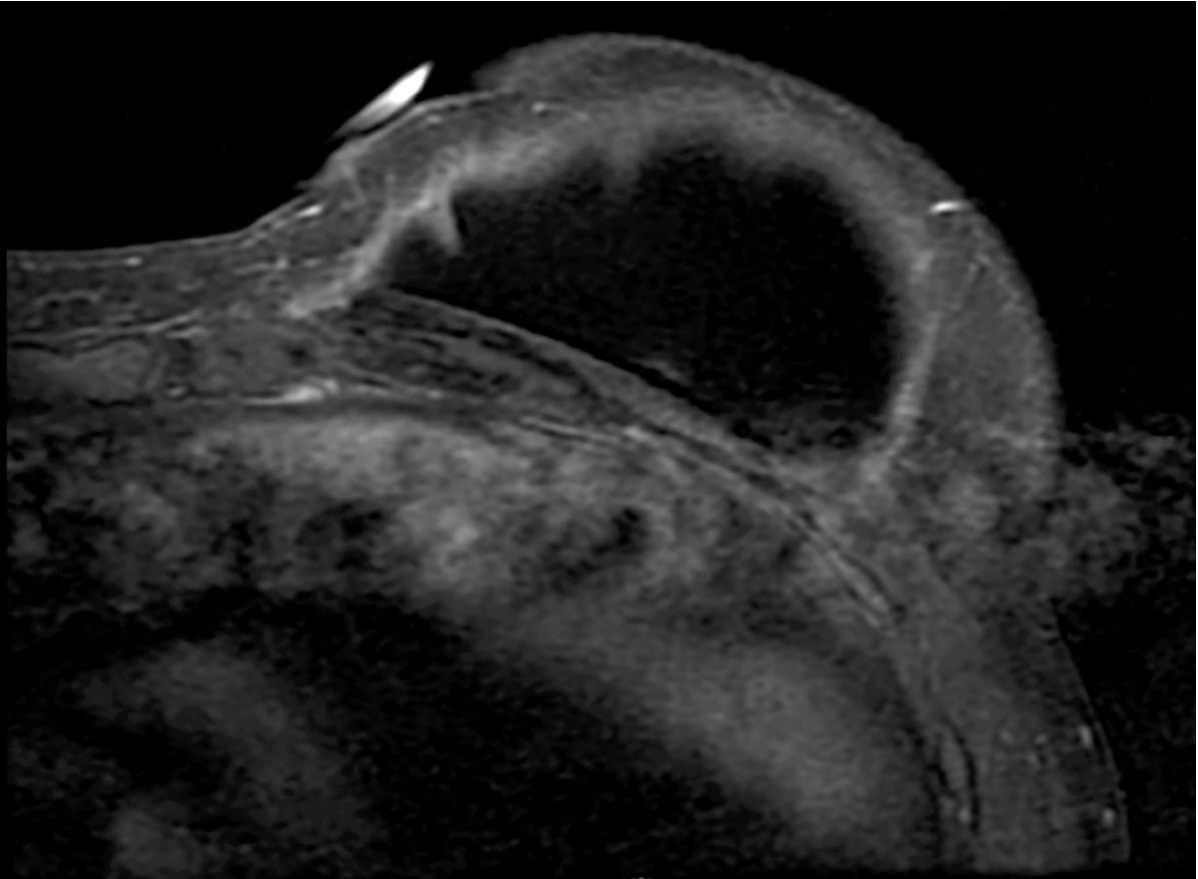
Follow up T1-weighted post-contrast MRI of the breast performed 9 months after the second cryoablation demonstrates reduction of size of desmoid tumor and intact breast implant.
